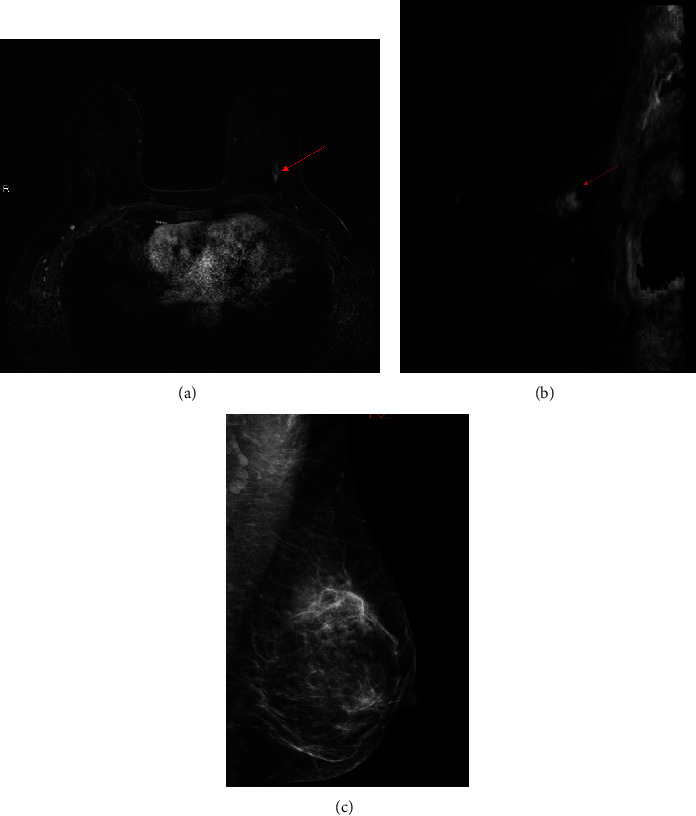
40 year-old BRCA1 patient undergoing annual MRI. Axial (a) and Sagittal (b) reconstruction showing a segmental nonmass enhancement in the left breast (red arrow). Mediolateral (MLO) view of mammography of the same breast shows no corresponding calcifications.
